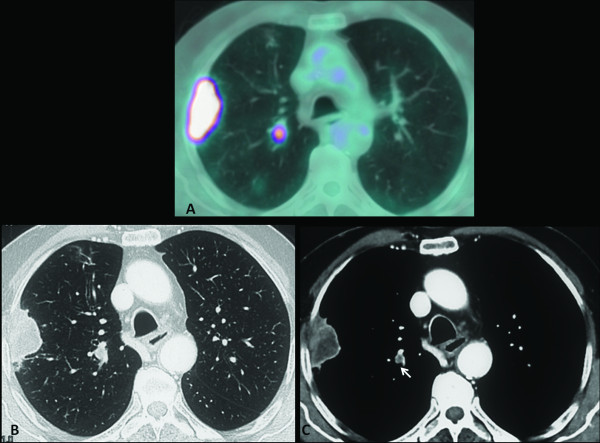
The not recognized nodule. (a) ^18^ F-FDG PET-CT fused axial image: intense ^18^ F-FDG uptake in the mass of the upper right lobe. Another focus of intense ^18^ F-FDG uptake was evident medially in the upper right lobe. (b, c) A retrospective analysis of contrast-enhanced CT revealed a small nodule located near vessels corresponding to the site of ^18^ F-FDG uptake (white arrow).
